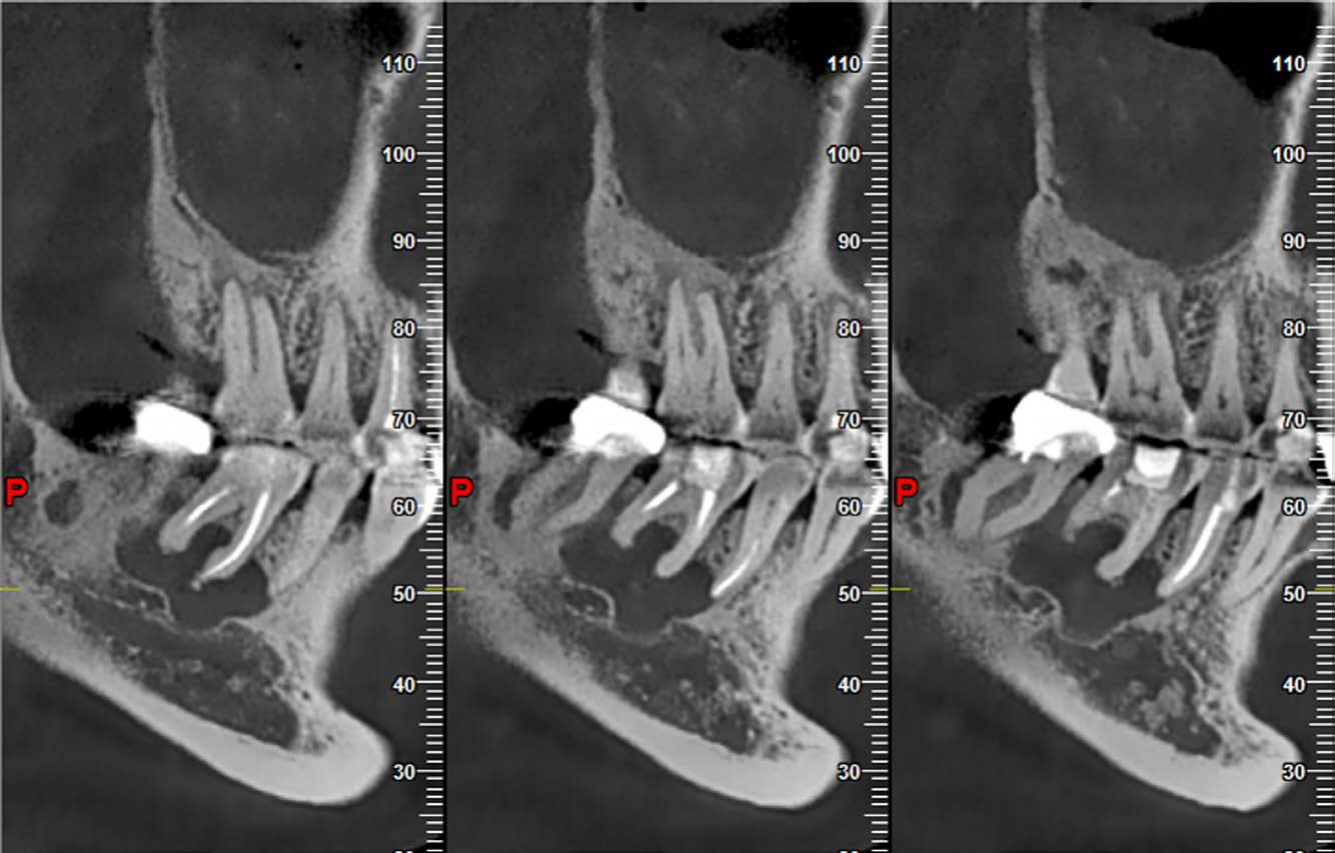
CBCT mesiodistal view: a thin sclerotic margin around the lesion, the shape is irregular with a shallow lobulated appearance, and it is adjacent to the mandibular canal below.
